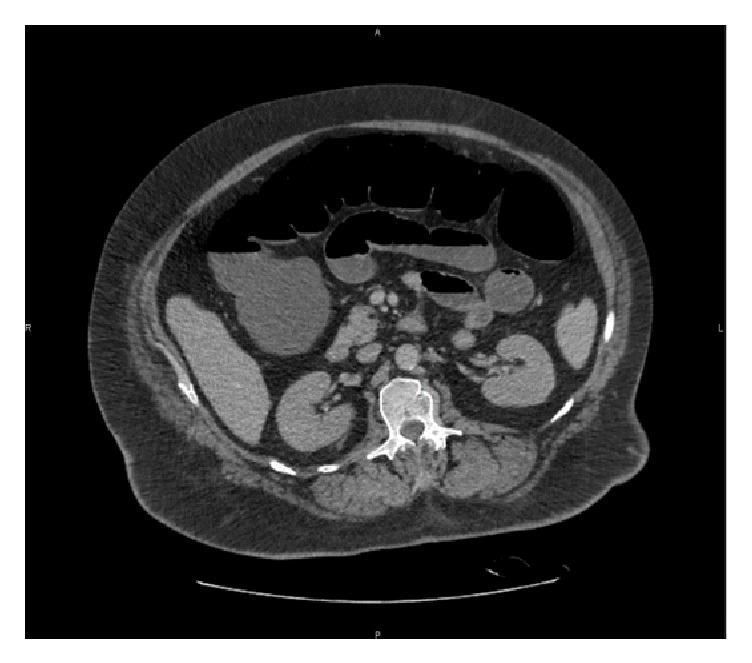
Follow-up abdomen computed tomography (CT) without evidence of mechanical obstruction, inflammatory or ischemic lesions.
